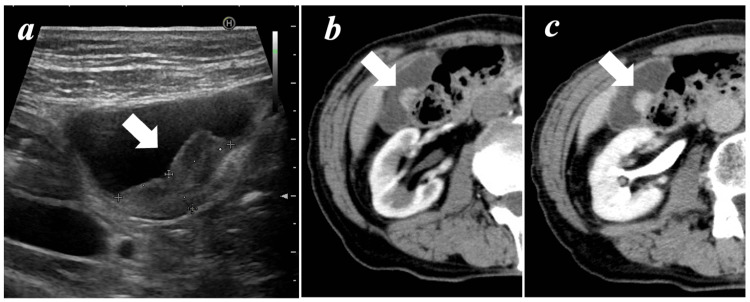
Abdominal ultrasonography (a); Abdominal tomography showing tumor in arterial phase (b) and venous phase (c) Abdominal ultrasonography revealed a well-defined, smooth mass of 22×8 mm inside the gallbladder (arrow) (a). Abdominal computed tomography showed a slightly enhanced tumor in the arterial phase (arrow) (b), however, there was no washout in the venous phase (c).
